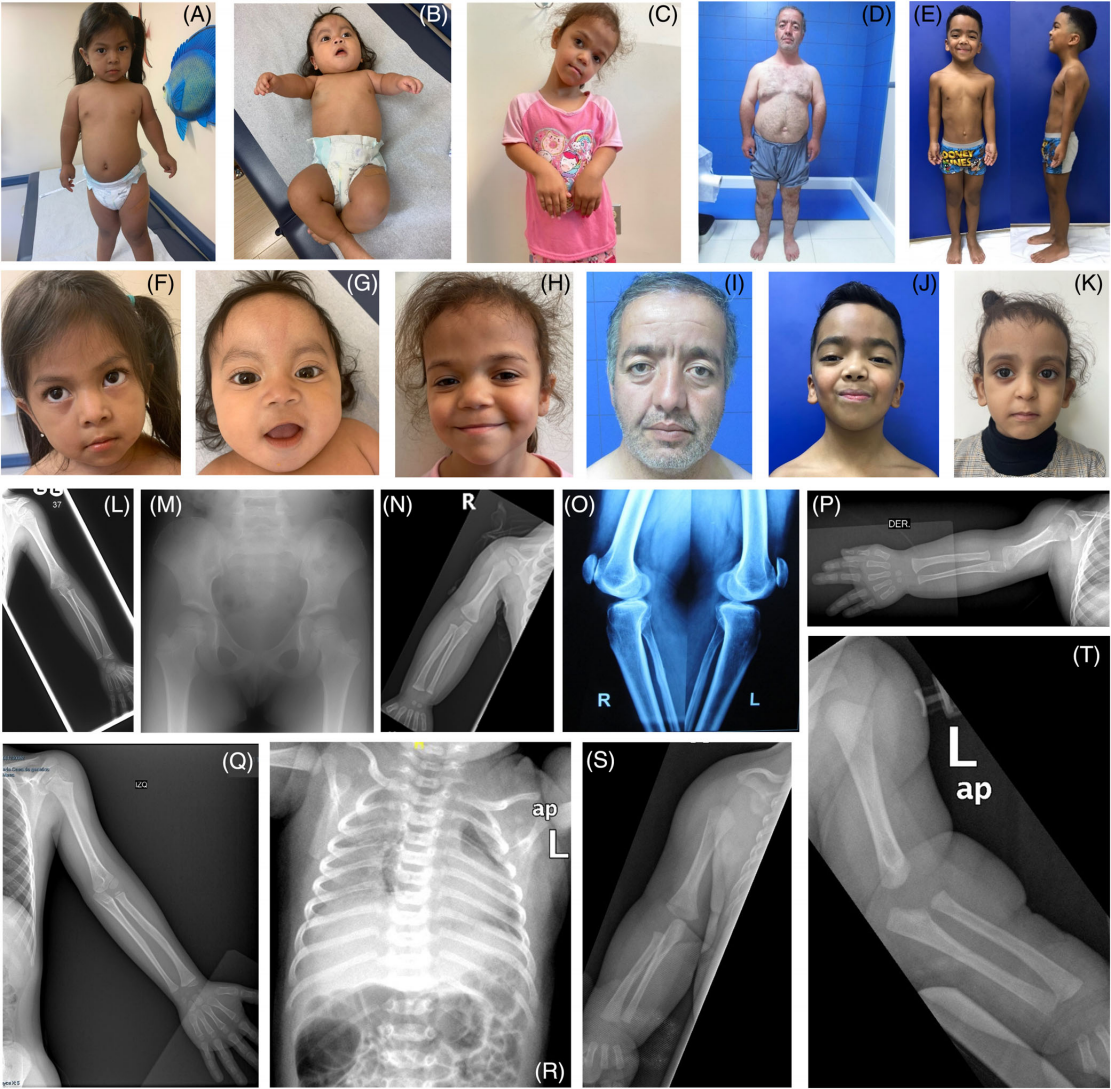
Clinical and radiographic images for individuals with biallelic *PKDCC* variants. Full length (A–E) and facial photos (F–K) of affected individuals from Families 3–7. (A, F) Photos showing F3‐II‐1 (aged 3 years) with rhizomelia of the upper limbs and widely spaced nipples. (B, G) Photos showing F3‐II‐2 (aged 4 months) with thick, arched, eyebrows, nevus flammeus, thin upper lip and a long philtrum. (C, H) Photos showing F4‐II‐2 (aged 5 years) with rhizomelia of the upper limbs, midface hypoplasia, a high forehead, hypertelorism, a thin upper lip and a long philtrum. (D, I) Photos showing F5‐II‐10 with high and broad forehead, flat face, rhizomelia of upper and lower limbs. (E, J) Photos showing F6‐II‐2 (aged 7 years 4 months) with prominent forehead, flat face, low set ears, sloping shoulders, pectus excavatum and rhizomelia. (K) Photo of F7‐II‐5 showing prominent eyes/forehead. (L) Antero‐posterior radiograph of left upper limb in F1‐II‐1 (aged 4 years 10 months). There is ‘mild shortening of the humerus’ (rhizomelia). (M) Antero‐posterior radiograph of pelvis in F1‐II‐1 (aged 4 years 10 months) showing ‘mild coxa valga’. (N) Antero‐posterior radiograph of the right upper limb of F2‐II‐1 (aged 9 months) demonstrating isolated ‘shortening of the humerus’ (rhizomelia). (O) Lateral knee radiographs of individual F5‐II‐10 show superior subluxation of the patellae and bilateral degenerative change with narrowing of the patellofemoral joints (worse on the right). (P, Q) Antero‐posterior radiograph of the upper limbs of F6‐II‐2 (aged 1 year and at 6 years 5 months) demonstrating ‘shortening of the humerus’ (rhizomelia). (R) AP chest and upper limb radiographs taken of F7‐II‐5 at birth show ‘11 ribs’ bilaterally and (S, T) ‘shortening of the humeri’ (rhizomelia). Radiographs annotated using terms from the dREAMS ontology (https://d‐reams.org; inverted commas). [Colour figure can be viewed at wileyonlinelibrary.com]
